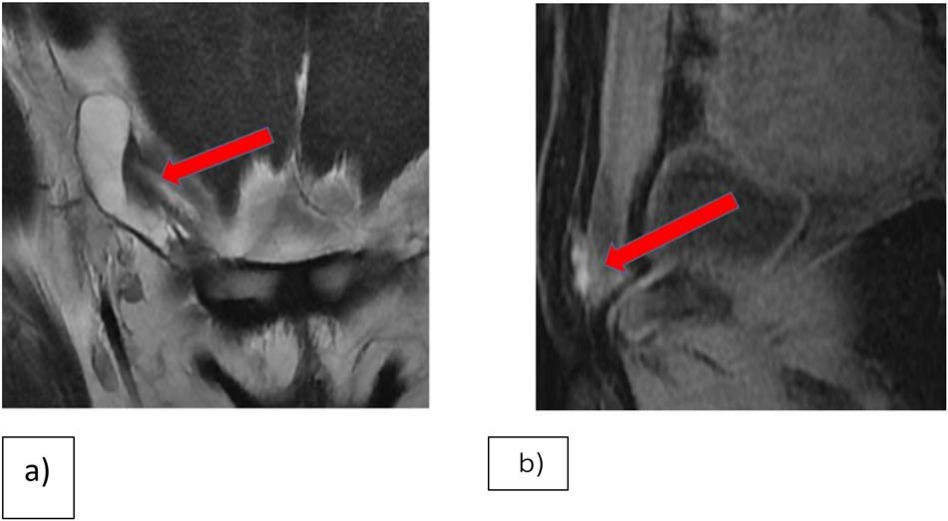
(a) Coronal T2WI showing right inguinal tubular cystic lesion that is communicating with peritoneum highly suggestive of communicating hydrocele of canal of Nuck. (b) MRI Sagittal T1 fat saturation, demonstrating that the medial superior aspect of the cyst is bright on T1WI in keeping with hemorrhagic contents versus endometrial implant.
